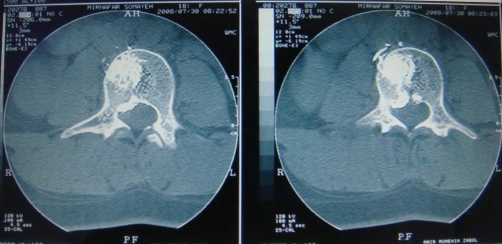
CT scan after vertebroplasty. Cement extrusion through the defect in posterior vertebral body is noted.
